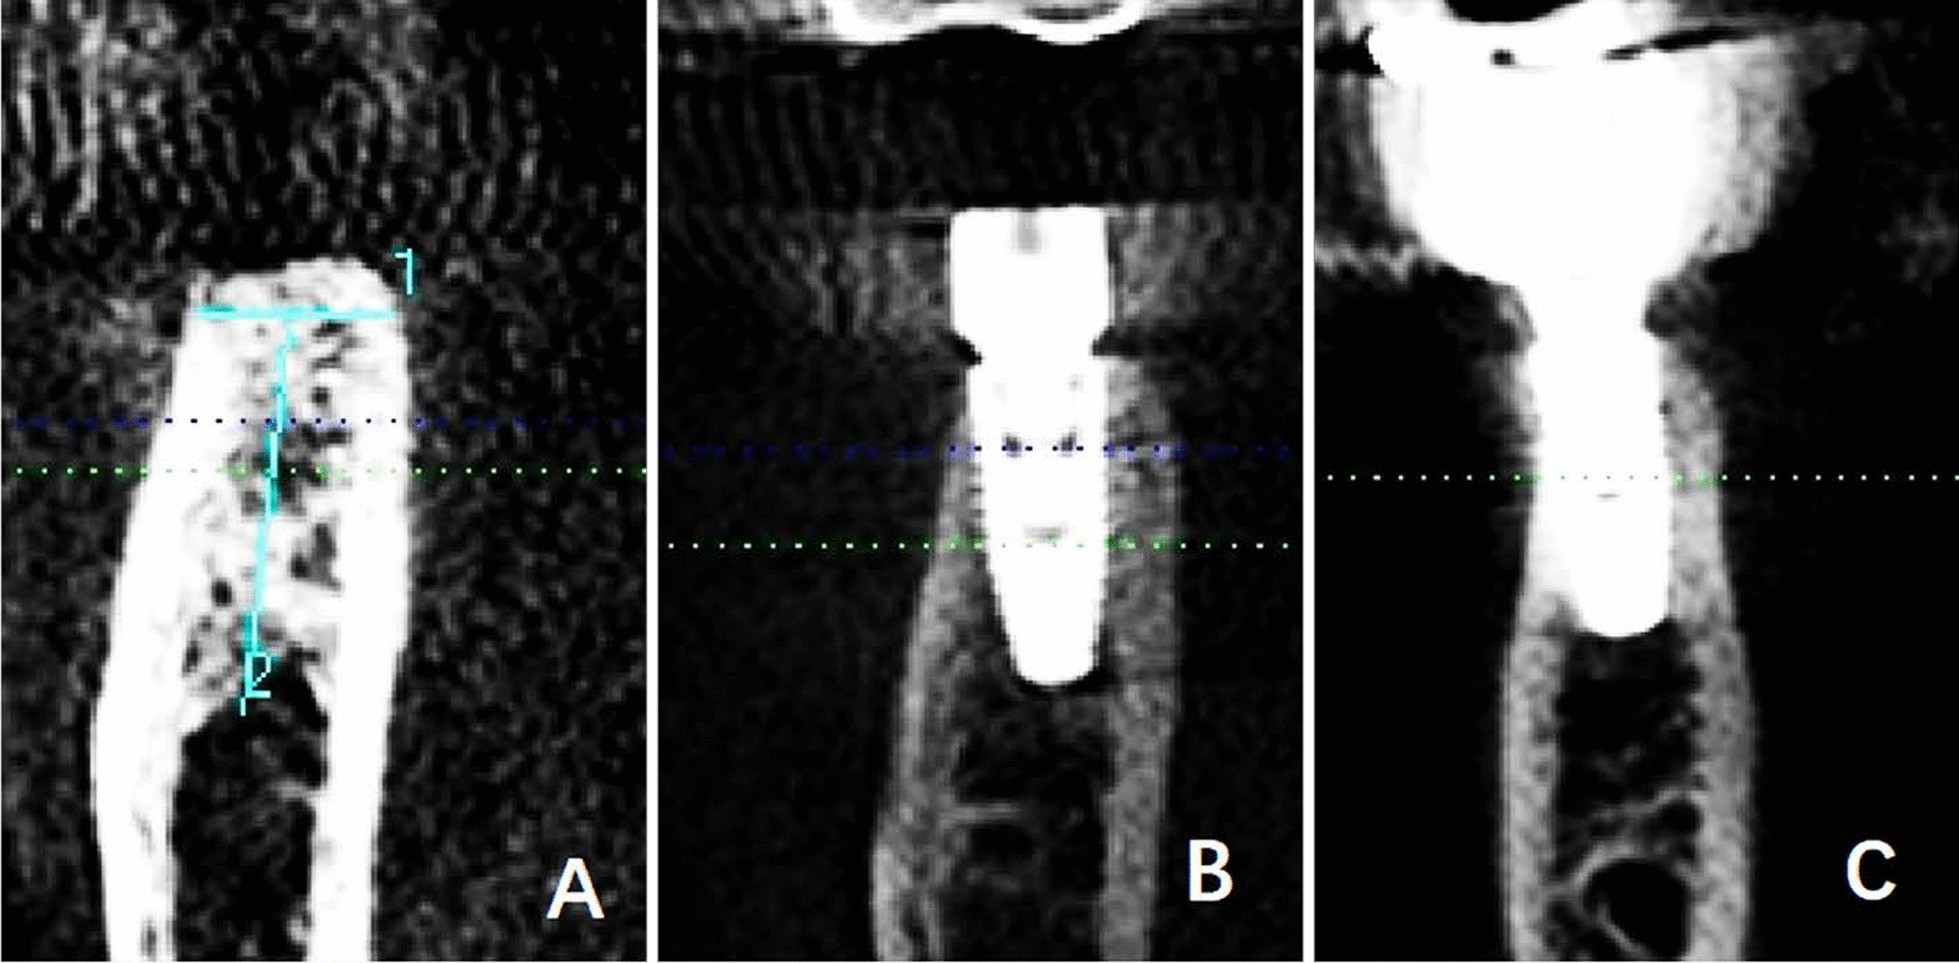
The placement of an implant in the straight type of cross-sectional posterior mandibular morphology. a The second subcategory of the straight type. b Cross-sectional CBCT image demonstrating dental implant insertion in the alveolar bone at the first molars. c Cross-sectional CBCT image after the prosthetic rehabilitation. A proper implant diameter should be decided based on the width of alveolar bone
